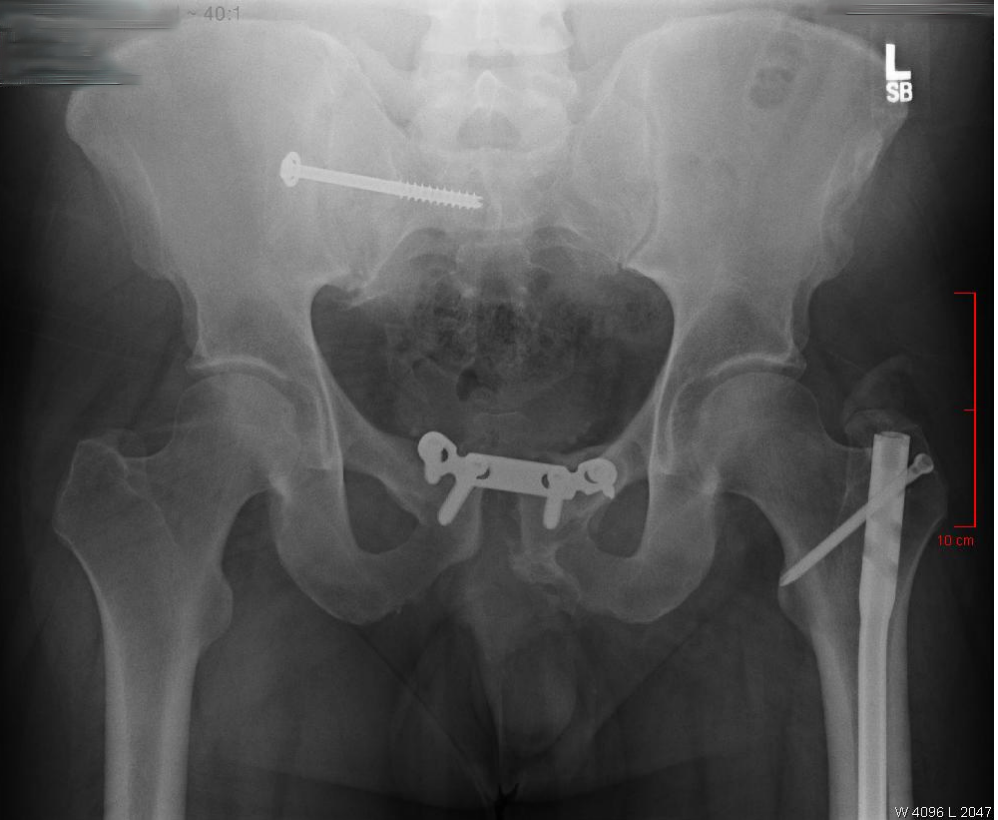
12 month follow-up AP pelvis radiograph showing mild loss of symphyseal reduction.
